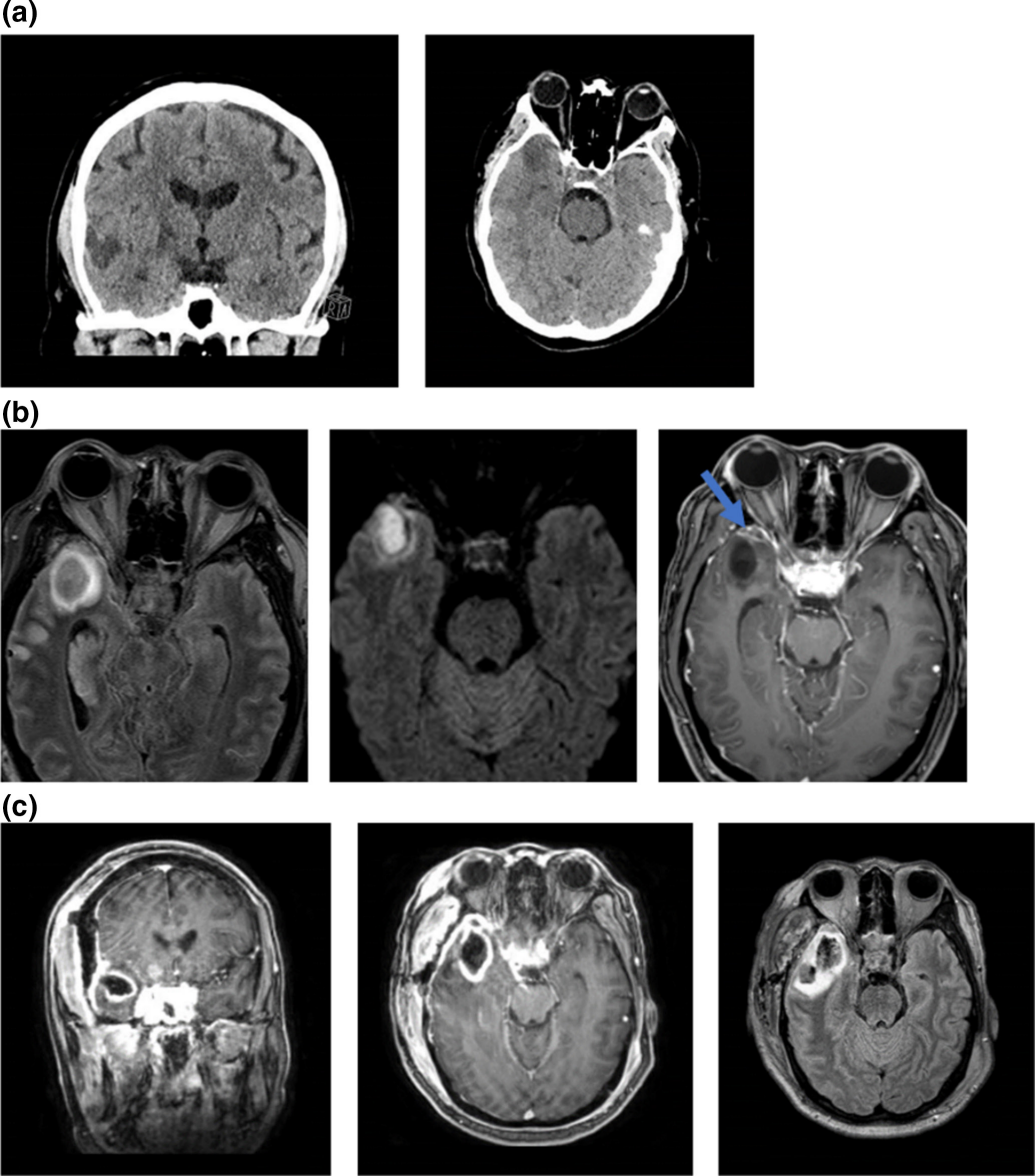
Pre- and post-operative neuroradiological studies identifying the brain abscess. (a) Axial (left) and coronal (right) non-contrast CT head demonstrate a hypodense 2.5 cm right anterior temporal lobe lesion. (b) Axial T2-FLAIR, DWI, and post-contrast T1 weighted images demonstrate a right anterior temporal cystic lesion with surrounding vasogenic oedema (left). There is central restricted diffusion (middle) and subtly peripheral enhancement (right, arrow) consistent with abscess. (c) Post-operative coronal and axial post-contrast T1 weighted images (left and middle) and axial T2-FLAIR images (right) demonstrate a post-operative hematoma in the right temporal lobe with persistent peripheral enhancement due to possible residual abscess.
